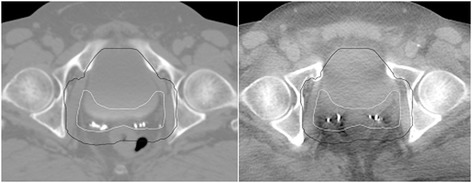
Increased rectum inside PTV. Geographic miss could be avoided using soft tissue matching in a number of instances. A planning CT (*left*) and CBCT scan (*right*) are shown in this example. In this case averaged soft tissue matching would have allowed a geographic miss to be corrected using the 1 cm uniform PTV expansion (*black line*), but a large amount of rectum would have been treated. The white line is the CTV. Critical structure avoidance therefore needs to be reviewed when matching online
